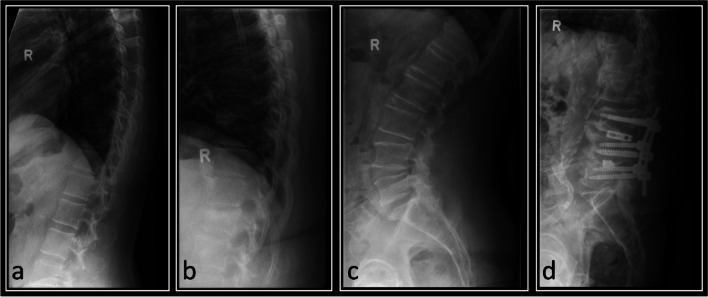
a–d From left to right: lateral radiographs of the thoracic (a, b) and lumbar spine (c, d) from different patients. Image a showing no mentionable degenerative changes, b showing mild changes, c moderate, and d severe changes
